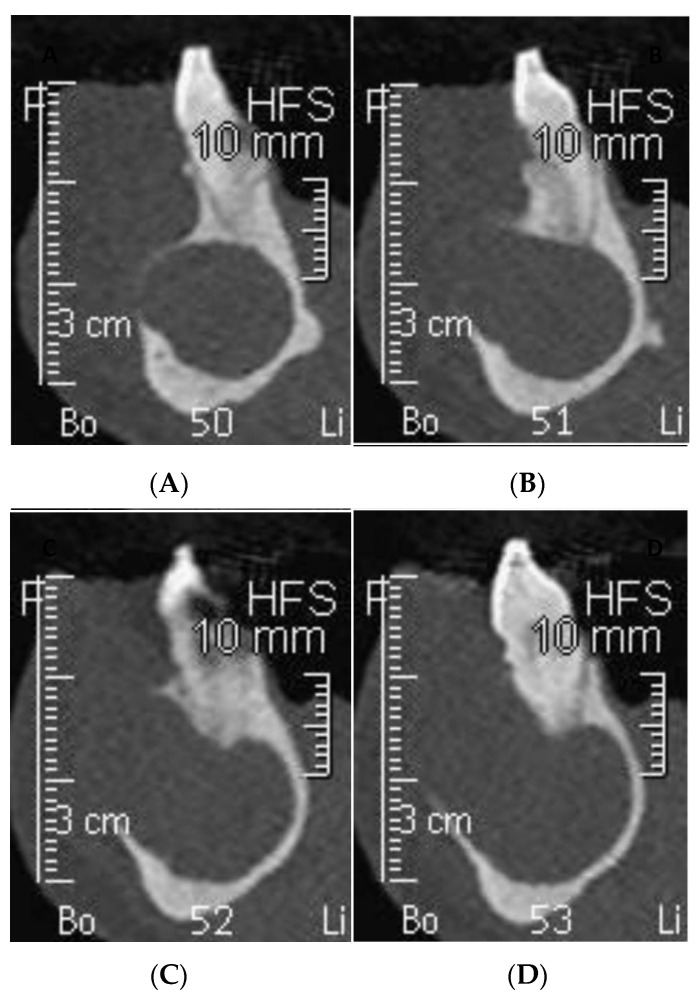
CBCT examination of sections between elements 3.3 and 3.4. (A) Distal aspect element 3.3 showing buccal osseous expansion. (B) Element 3.3, with evident buccal cortical bone erosion. (C) Increasing cortical opening due to cystic expansion. (D) Mesial of element 3.4 showing degree of buccal cortical bone elimination.
